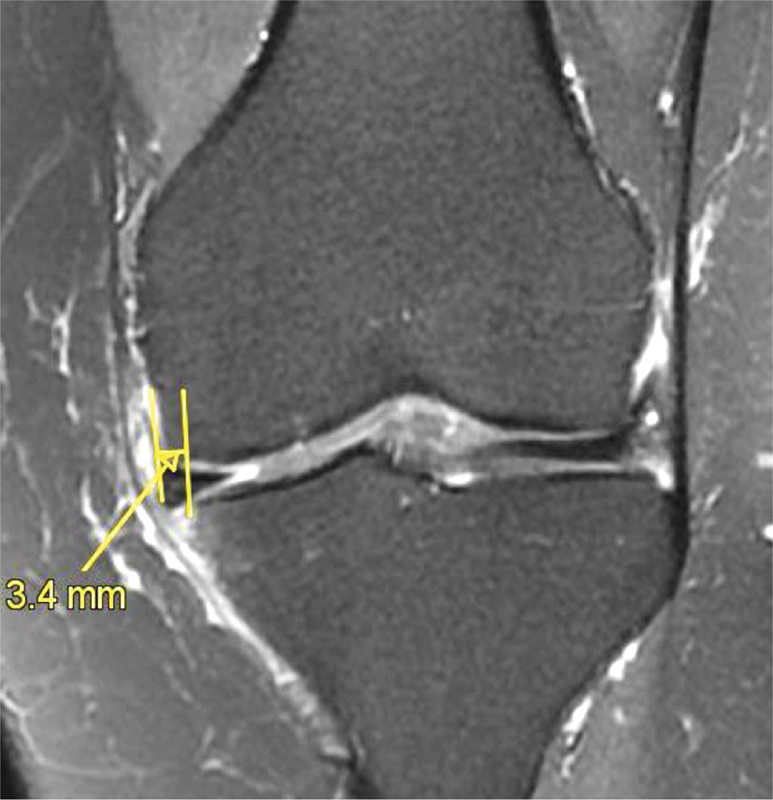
Medial meniscus extrusion (>3 mm) demonstrating an early osteoarthritic stage (MRI: coronal view; T2 FS).
